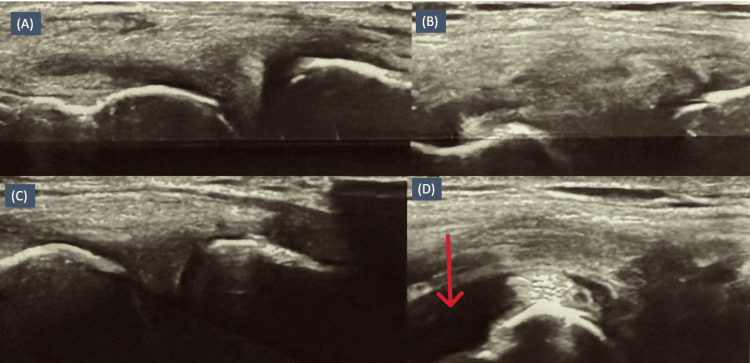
Ultrasonography of the left knee demonstrating mild joint effusion predominantly within the suprapatellar recess (arrow).
